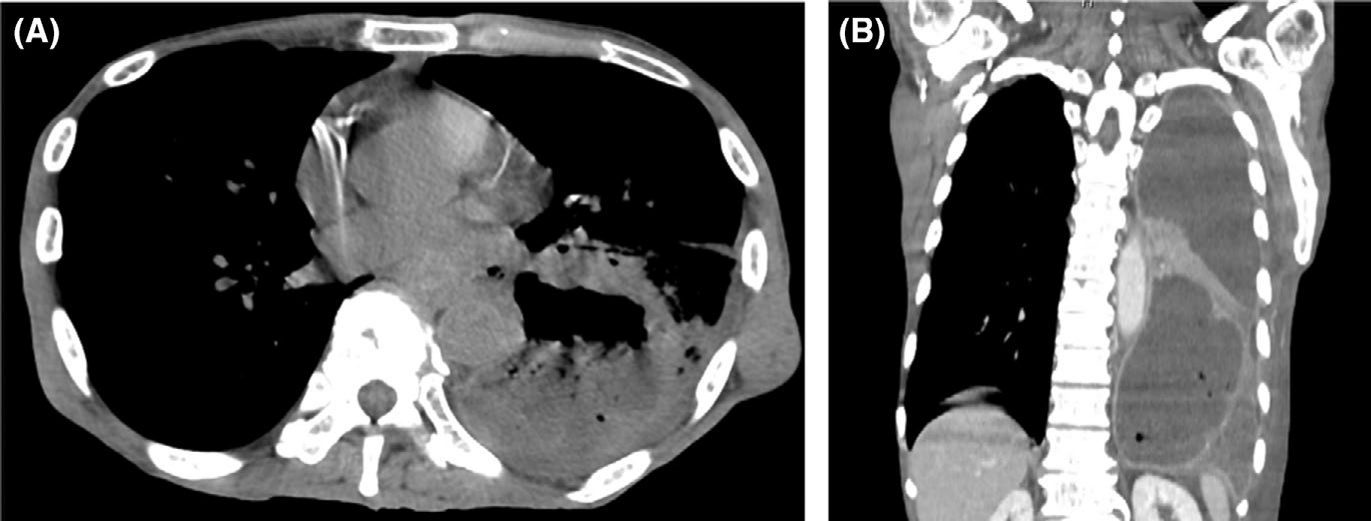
Non‐contrast chest CT images (A) obtained at admission revealed a large lung abscess in the left lower lobe accompanied by pneumothorax. Subsequent contrast‐enhanced chest CT images (B) provided clearer delineation of the abscess and demonstrated its rupture, resulting in empyema.
